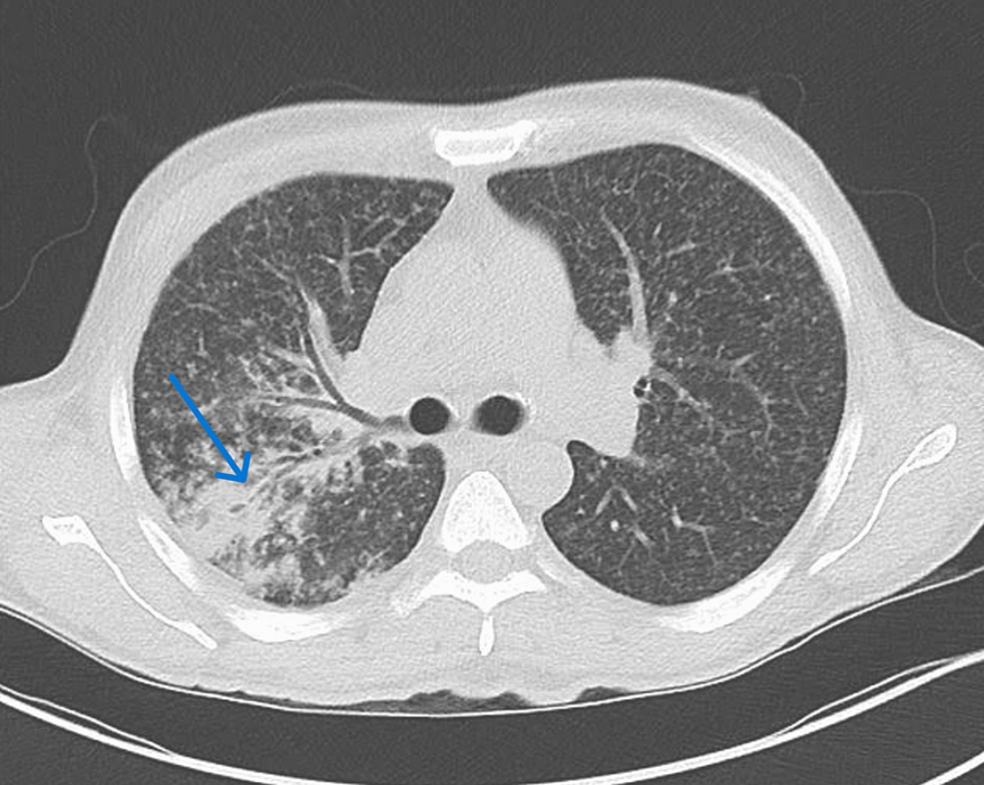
A CT scan of the lung showing irregular consolidation in the right upper lobe (blue arrow)
